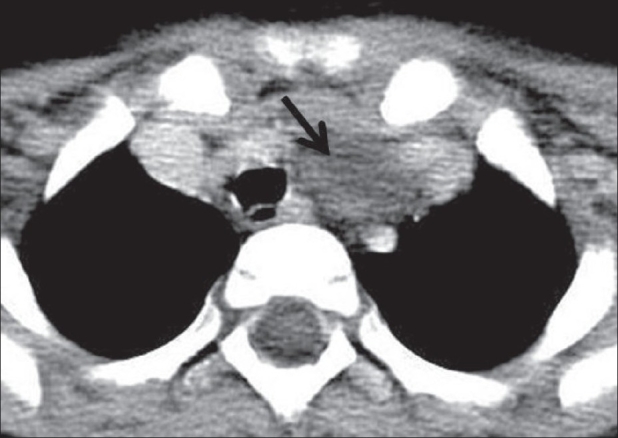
Axial CT scan of the neck shows the caudal (distal) end of the lesion (arrow), extending into the superior mediastinum, and its relation to the thymus
